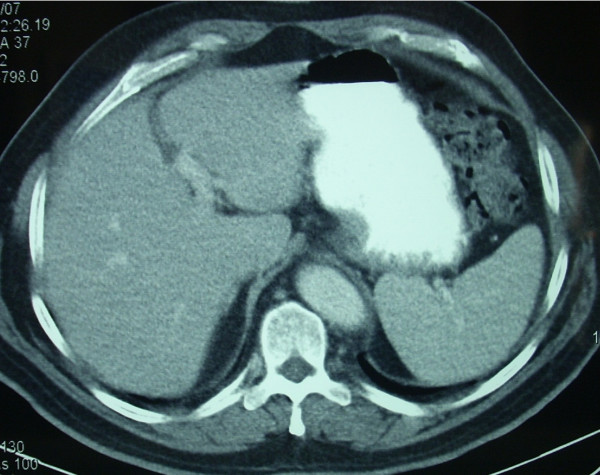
Clinical complete response assesed in thoracic-abdoninal-pelvic CT performed in May 2007, after neoadjuvant systemic chemotherapy.
